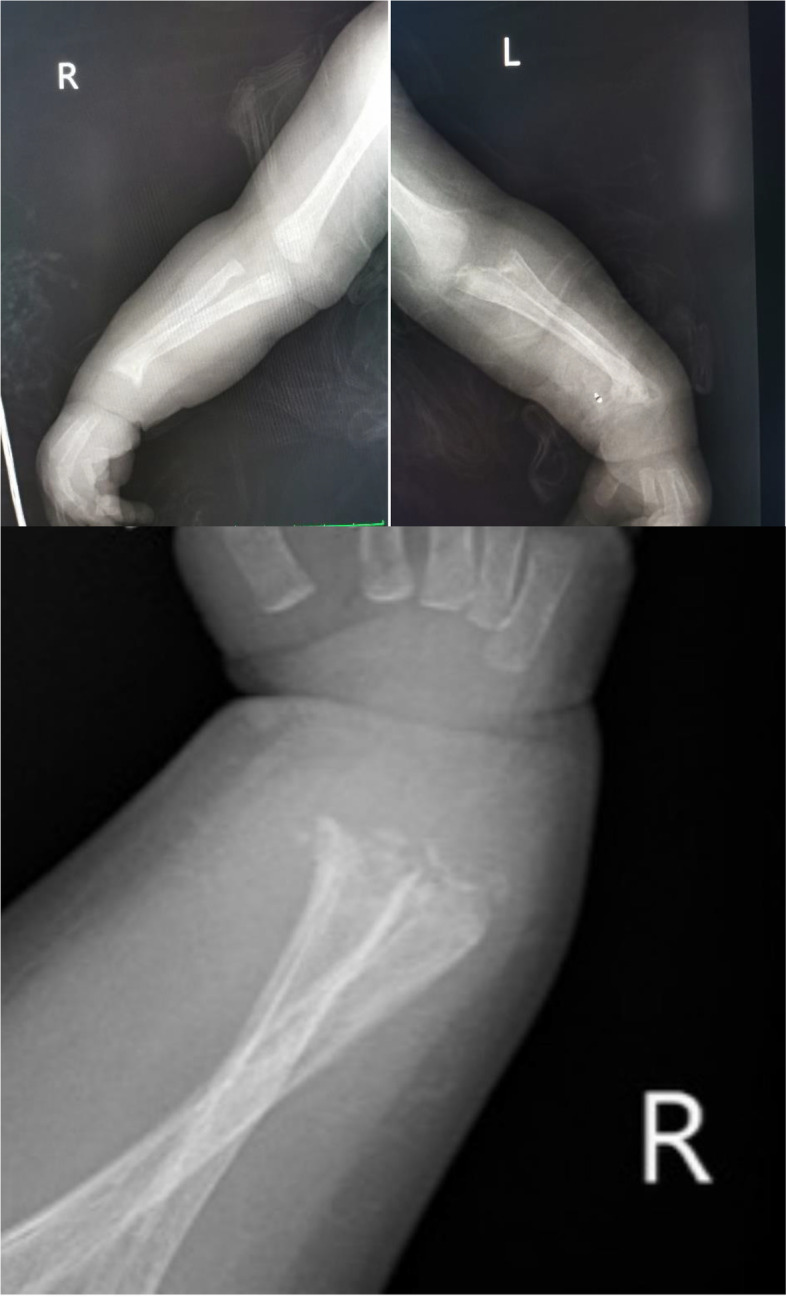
X-ray findings of long bones
